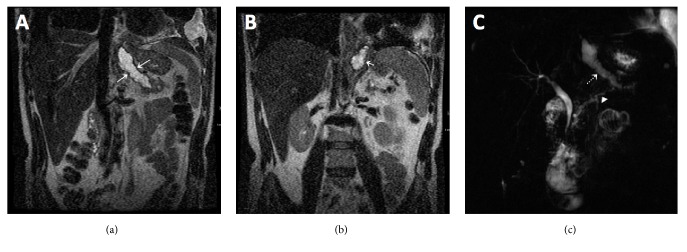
Coronal T2-weighted MRCP images (a, b) demonstrate a hyperintense tract arising from the tail of pancreas ((a) large white arrows), crossing the diaphragm and entering the pleural space ((b) short white arrow). Thick slab 2D MRCP images (c) show an irregular main pancreatic duct (white arrow head) and redemonstrates the fistula tract arising from the tail of pancreas (white dashed arrow).
